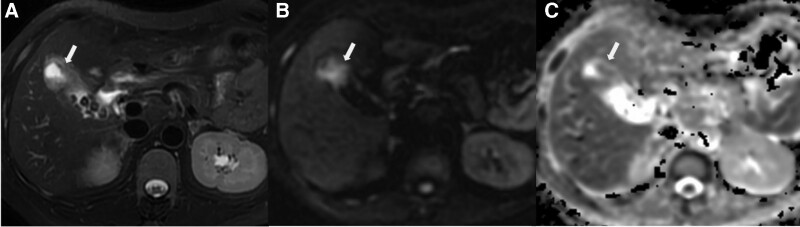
Moderately-poorly differentiated gallbladder adenocarcinoma in a 48-year-old female. (a) Focal wall thickening without layered appearance in the fundus of gallbladder (arrow) was shown on T2WI fat saturation image (type 3), with papillary growth pattern; (b, c) Localized hyperintensity (arrow) was shown on DWI and hypointensity (arrow) on ADC map, without layered pattern (type 3). DWI = diffusion-weighted imaging, T2WI = T2-wighted imaging.
